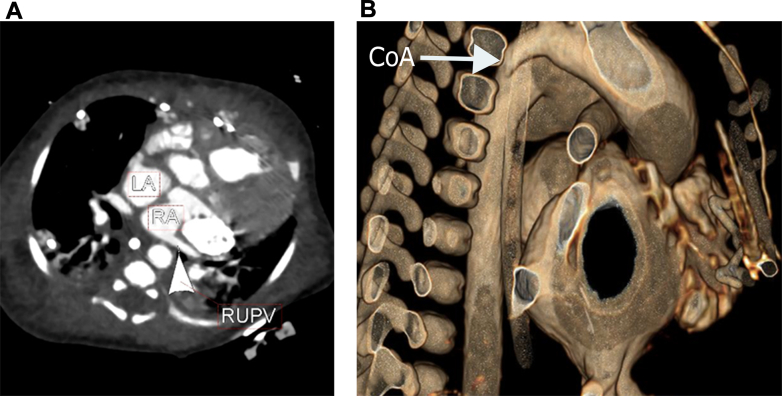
Computed Tomography Angiogram Demonstrating Venous and Arterial Anomalies (A) Computed tomography angiogram axial showing the right upper pulmonary vein (RUPV) connecting to the right atrium (RA) and (B) maximum-intensity projection reconstruction of the aortic arch showing the hypoplastic aortic isthmus (arrow). CoA = coarctation of aorta; LA = left atrium.
